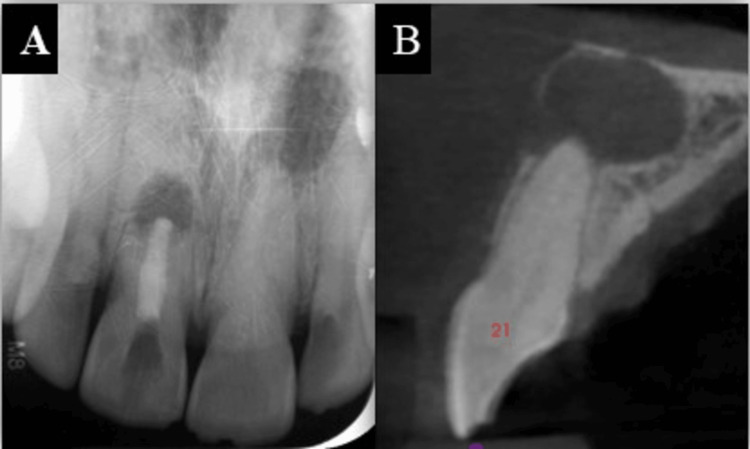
(A) Pre-operative radiograph and (B) pre-operative CBCT image CBCT: Cone beam computed tomography
